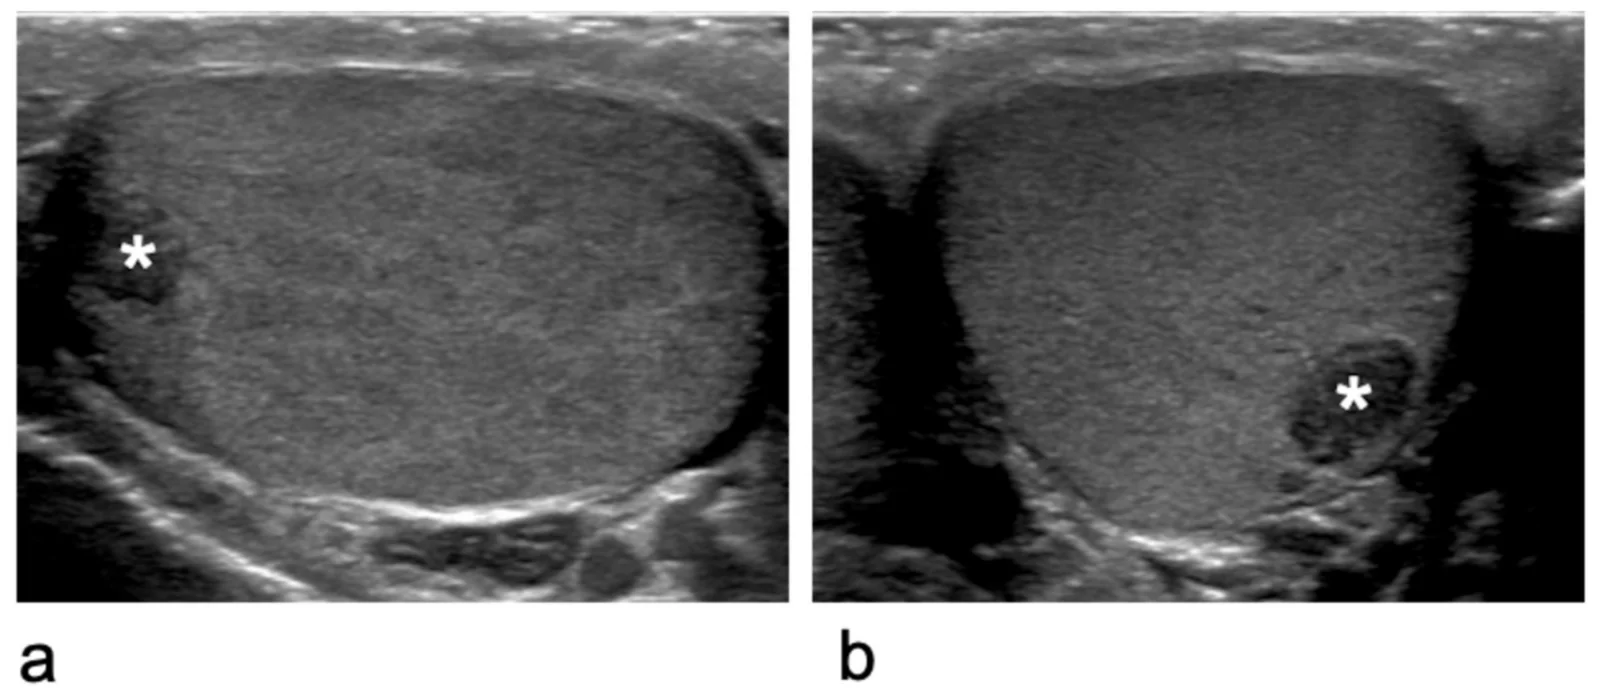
A 26-year-old man with congenital adrenal hyperplasia (CAH). Ultrasound shows bilateral testicular involvement by hypoechoic testicular adrenal rest tumours (TARTs), visible in the right (a) and left (b) testes (asterisks) near the rete testis.
